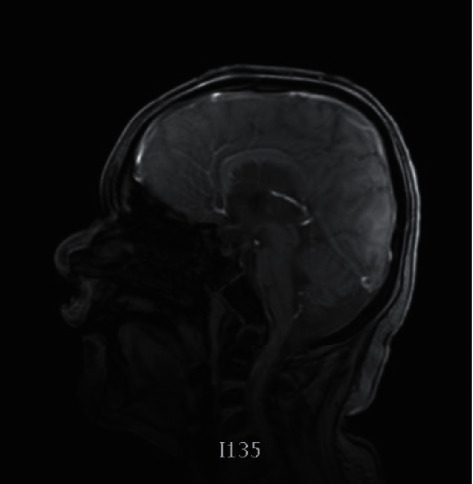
Brain MRI revealing dysgenesis of the corpus callosum, partially empty sella, and third ventricular enlargement.
